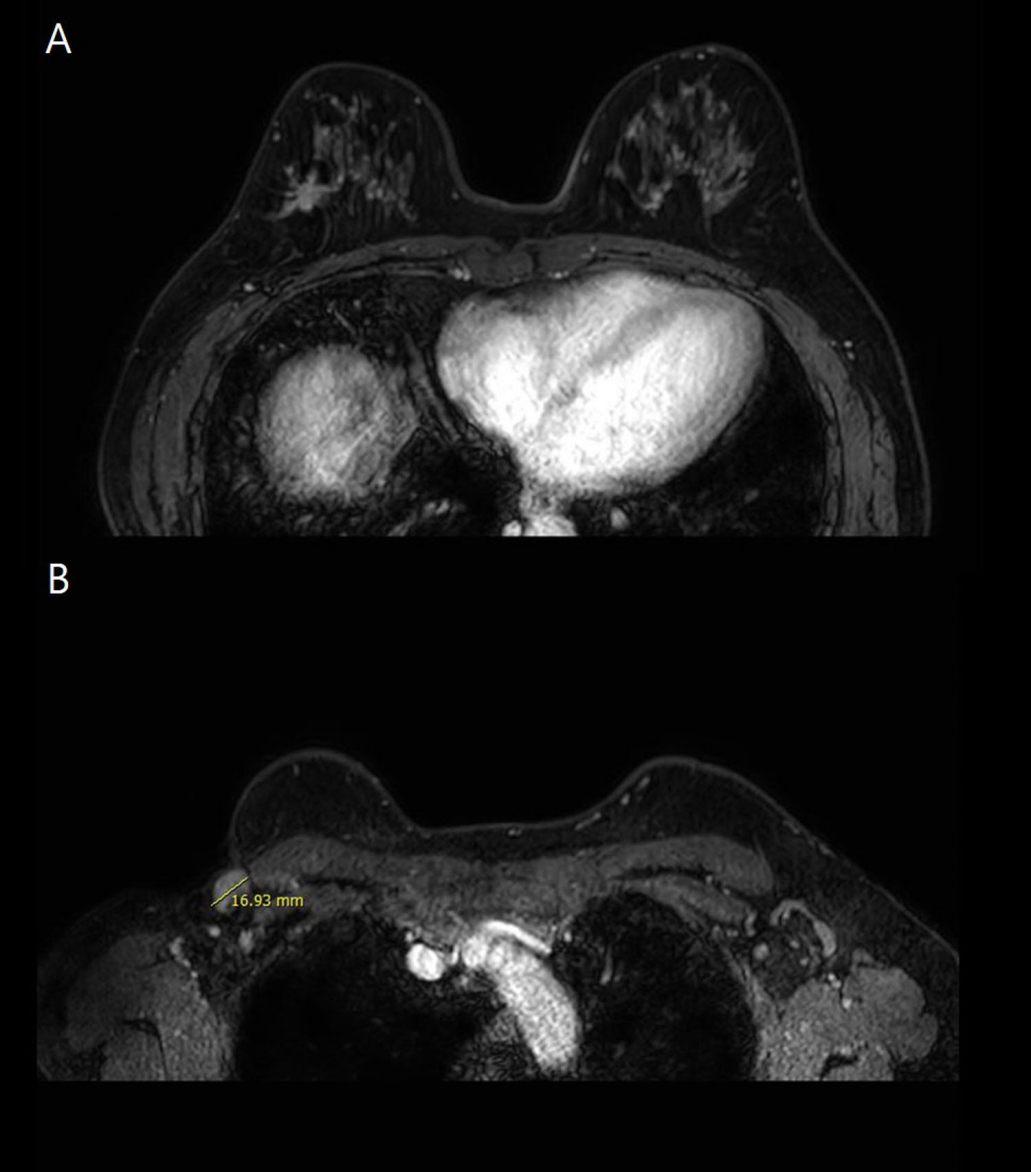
Breast MRI image of (A) breast and (B) axillary area.
